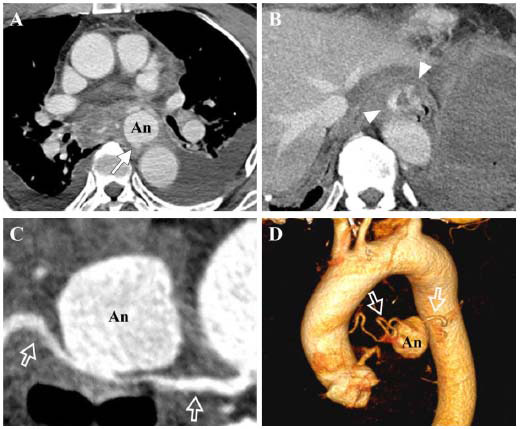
Contrast-enhanced computed tomography images in the venous phase of a 71-year-old man presenting with hematemesis. (A) A saccular aneurysm (An), approximately 3 cm in size, is seen in the posterior mediastinum with contrast extravasation through the posterior wall, suggesting a rupture (arrow). (B) Extravasated contrast medium is seen within the esophageal lumen (arrowheads). (C) Reconstructed image of the right bronchial artery (open arrows) using the centerline method shows connection with the aneurysm (An), indicating bronchial artery aneurysm. (D) Volume Rendering Technique (VRT) image clearly demonstrates the origin of the aneurysm (An) and the right bronchial artery (open arrows) from the thoracic aorta.
